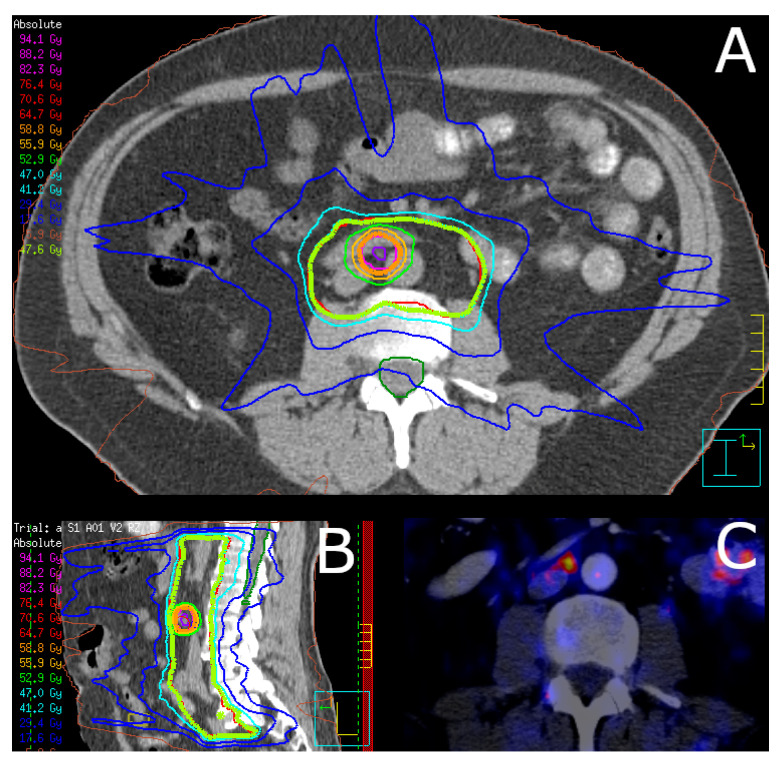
Exemplary dose distribution of salvage nodal radiotherapy. Shown is an exemplary dose distribution of a patient receiving PSMA PET-guided salvage nodal radiotherapy in (A) axial view and (B) sagittal view. The corresponding ^68^Ga-labeled PSMA PET shows a single paraaortic lymph node metastasis (C). The patient received volumetric modulated arc therapy with a simultaneous integrated boost to the involved PET-positive lymph node. Twenty-eight fractions with 1.7 Gy per fraction for the PTV and 2.1 Gy per fraction for the PTV_Boost_ were prescribed, resulting in total doses of 47.6 Gy and 58.8 Gy, respectively.
